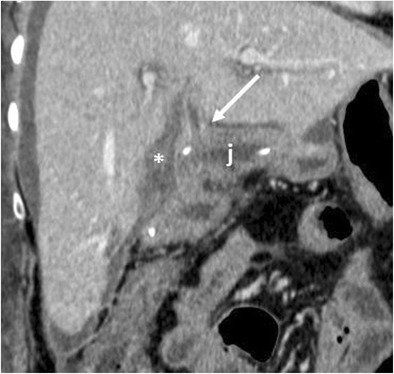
Biliary fistula. Coronal oblique CT image shows the biliary anastomosis (white arrow) surrounded by an ill-defined fluid collection (*). The jejunal loop is visible (j)
